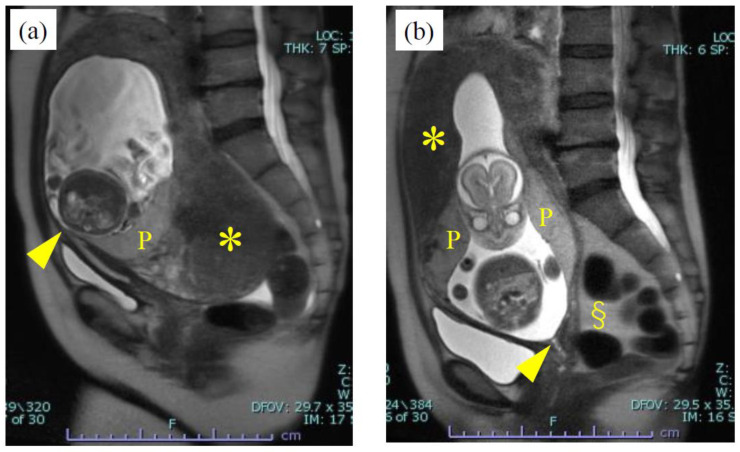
Sagittal T2-weighted MR images of Case 2. An enlarged uterine fundus with adenomyosis was entrapped within the pelvic cavity, and the cervix was stretched cranioventrally at 20 weeks of gestation (a). However, at 21 weeks, spontaneous resolution was confirmed with the finding of a normal position of the cervix (b). The arrowhead indicates cervical internal ostium. P, placenta; §, the amount of ascites was relatively larger than the normal physiological amount during pregnancy; * adenomyosis.
